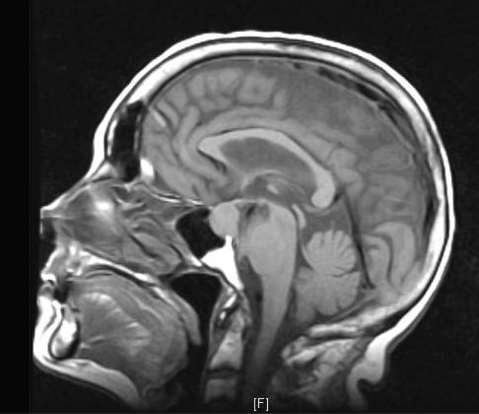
Sagittal view of head MRI T1 showing the pituitary macroadenoma.
